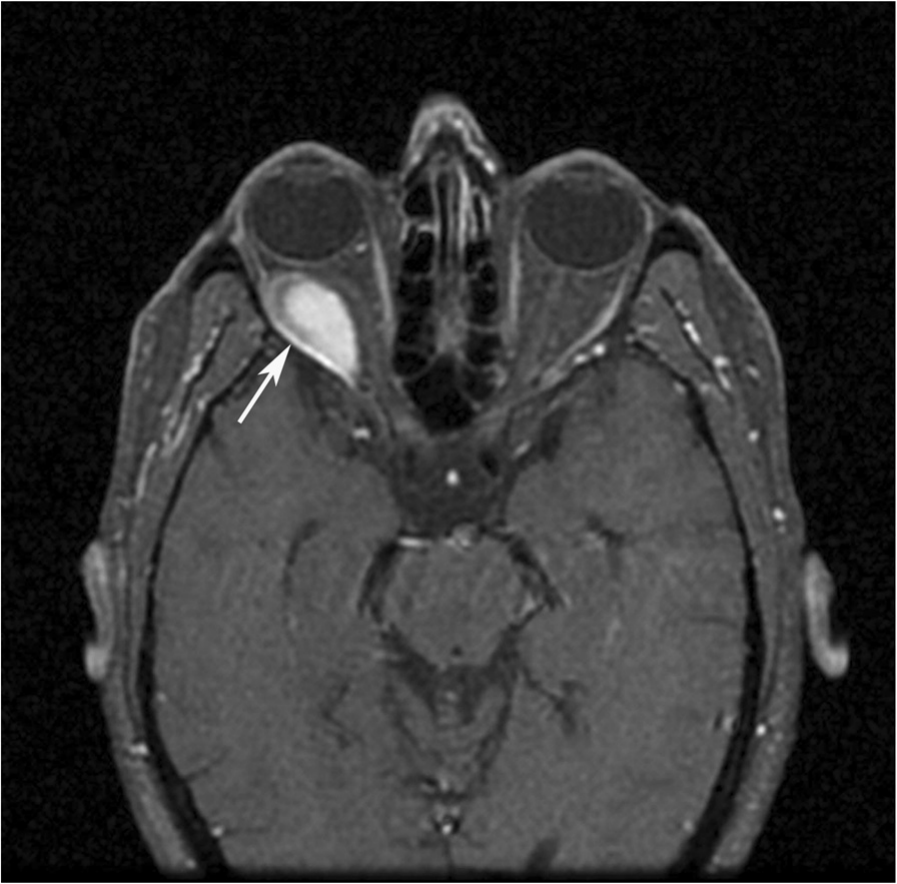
Axial fat sat T1-weighted MRI which showed ovoid shaped hyperintensity at right retrobulbar-extraconal region consistent with subperiostal haematoma.
